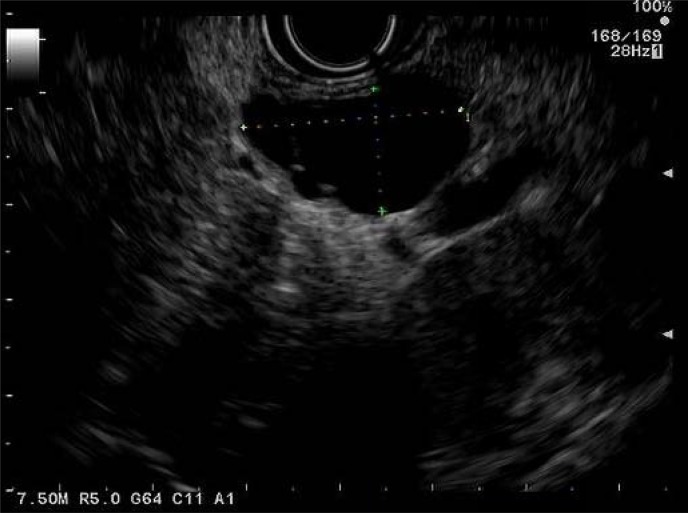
Endoscopic ultrasound image of a 2.3 × 1.3 cm anechoic lesion adjacent to the pancreatic tail.
